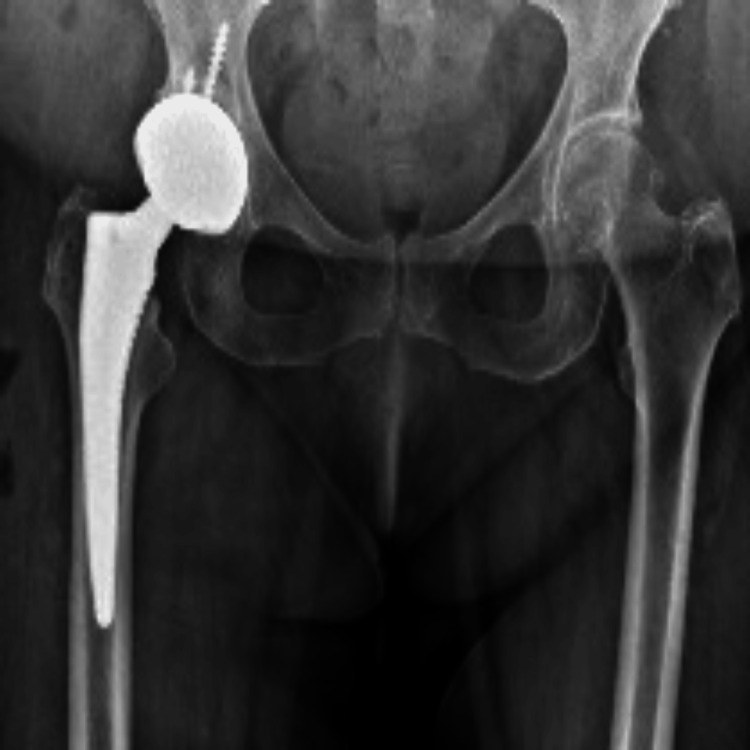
Postoperative plain radiograph
